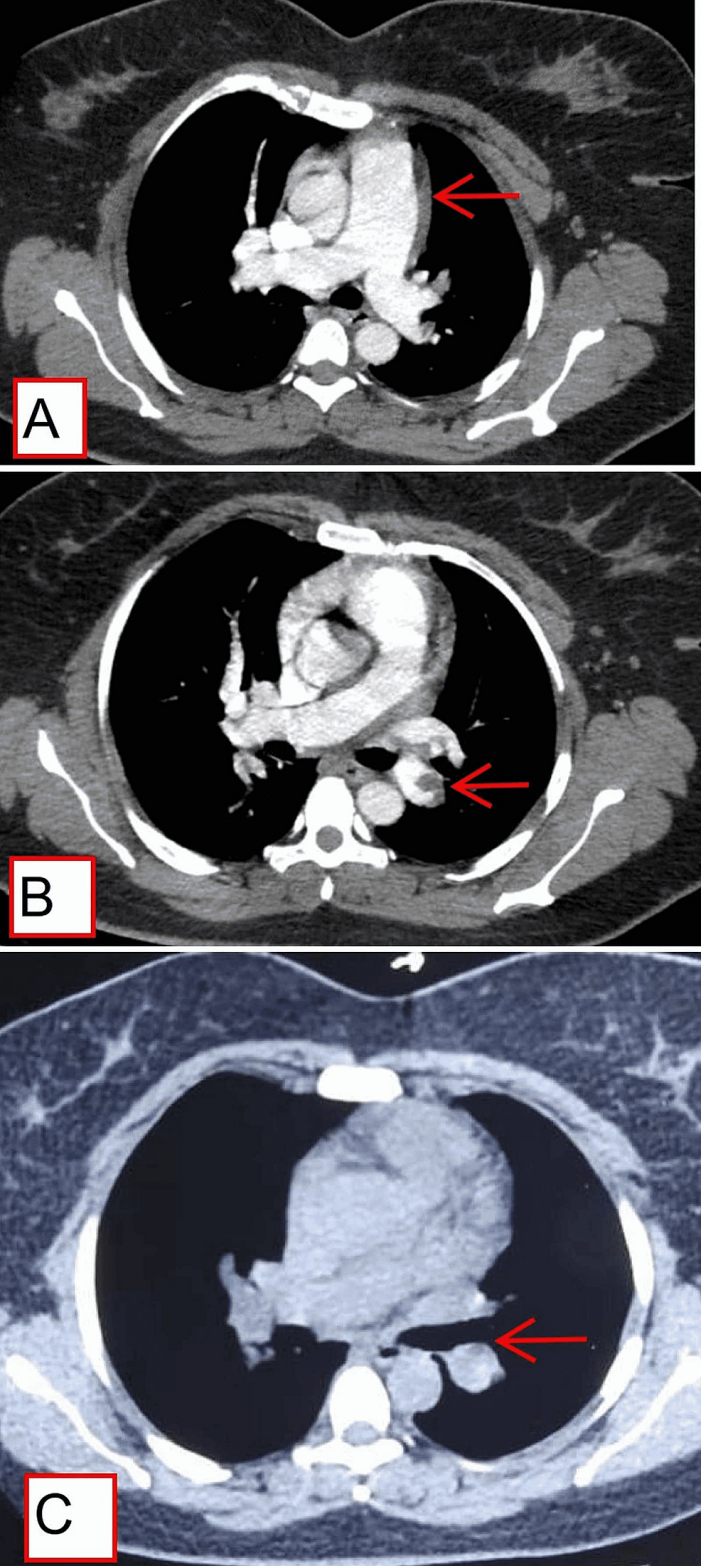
CT angiography and CT thorax findings (A) CT angiography shows dilated main pulmonary artery, (B) CT angiography shows filling-defect in the left descending pulmonary artery, and (C) non-contrast computed tomography (NCCT) thorax showing hypodensity in left pulmonary artery.
